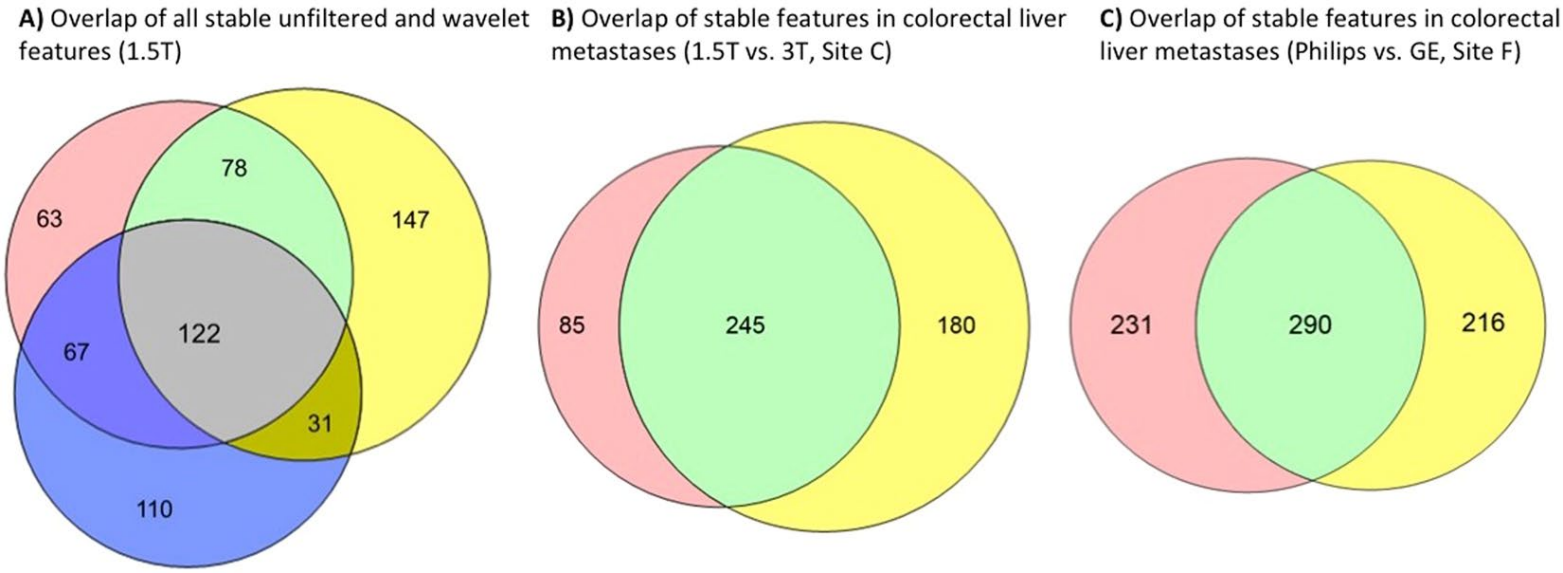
Overlapping results in feature stablility extracted from 1.5 T-MR images of (A) all tumour-entities (i.e., colorectal liver metastases (red), ovarian cancer (yellow), and lung cancer (blue)); derived from MR images of colorectal liver metastases (B) acquired at 1.5 T (red) and 3 T (yellow); and obtained from 3 T-MR images of colorectal liver metastases (C) acquired on a Philips Ingenia (red) and GE Discovery (yellow).
